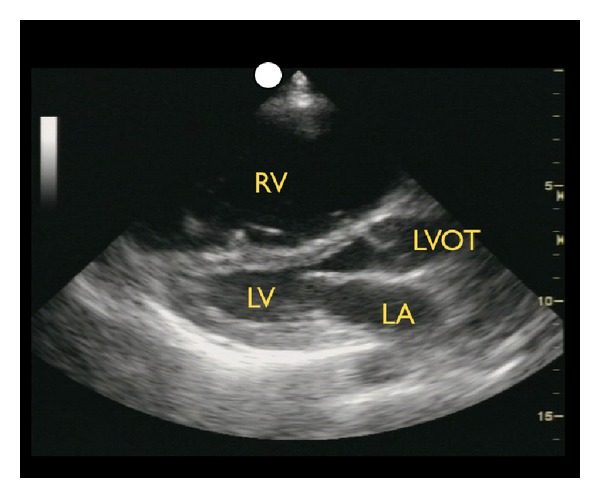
Right ventricular dilation, parasternal long axis view. RV: right ventricle, LA: left atrium, LV: left ventricle, LVOT: left ventricle outflow tract.
